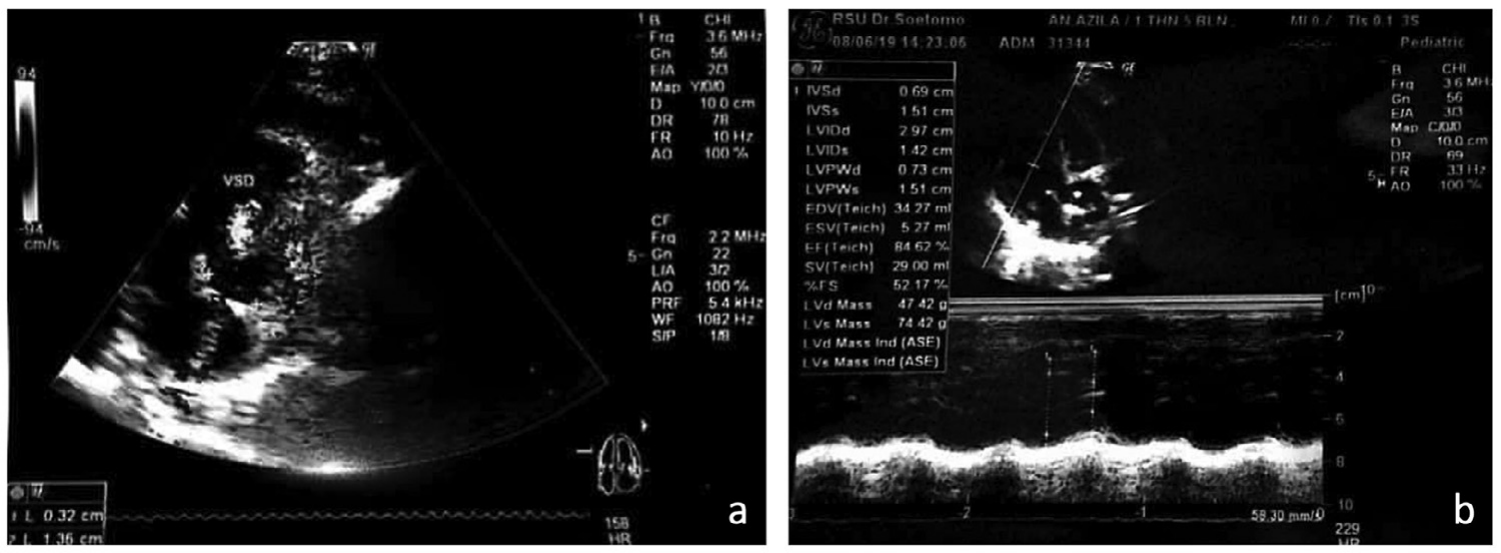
Echocardiography performed on August 6, 2019. (a) In the right baby, a small perimembranous VSD with diameter ± 0,32 cm was detected; (b) the left baby had normal echocardiography results.
